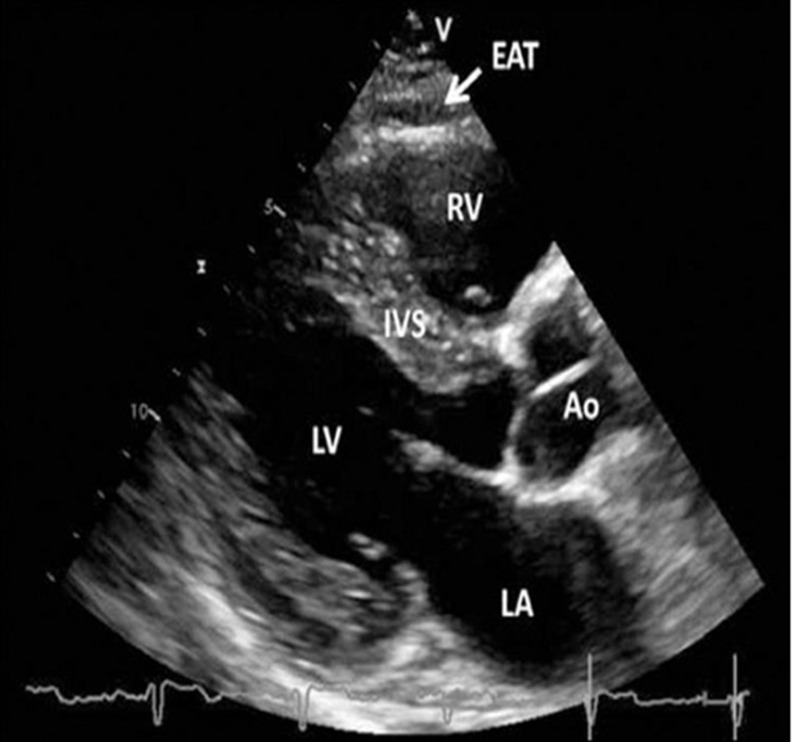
Transthoracic echocardiographic view of epicardial adipose tissue. Epicardial adipose tissue is an echo-lucent area between the epicardial surface and parietal pericardium in front of the right ventricular free wall and is indicated with a white arrow. Abbreviations: Ao, aorta; EAT, epicardial adipose tissue; IVS, interventricular septum; LA, left atrium; LV, left ventricle; RV, right ventricle [36, 37].
